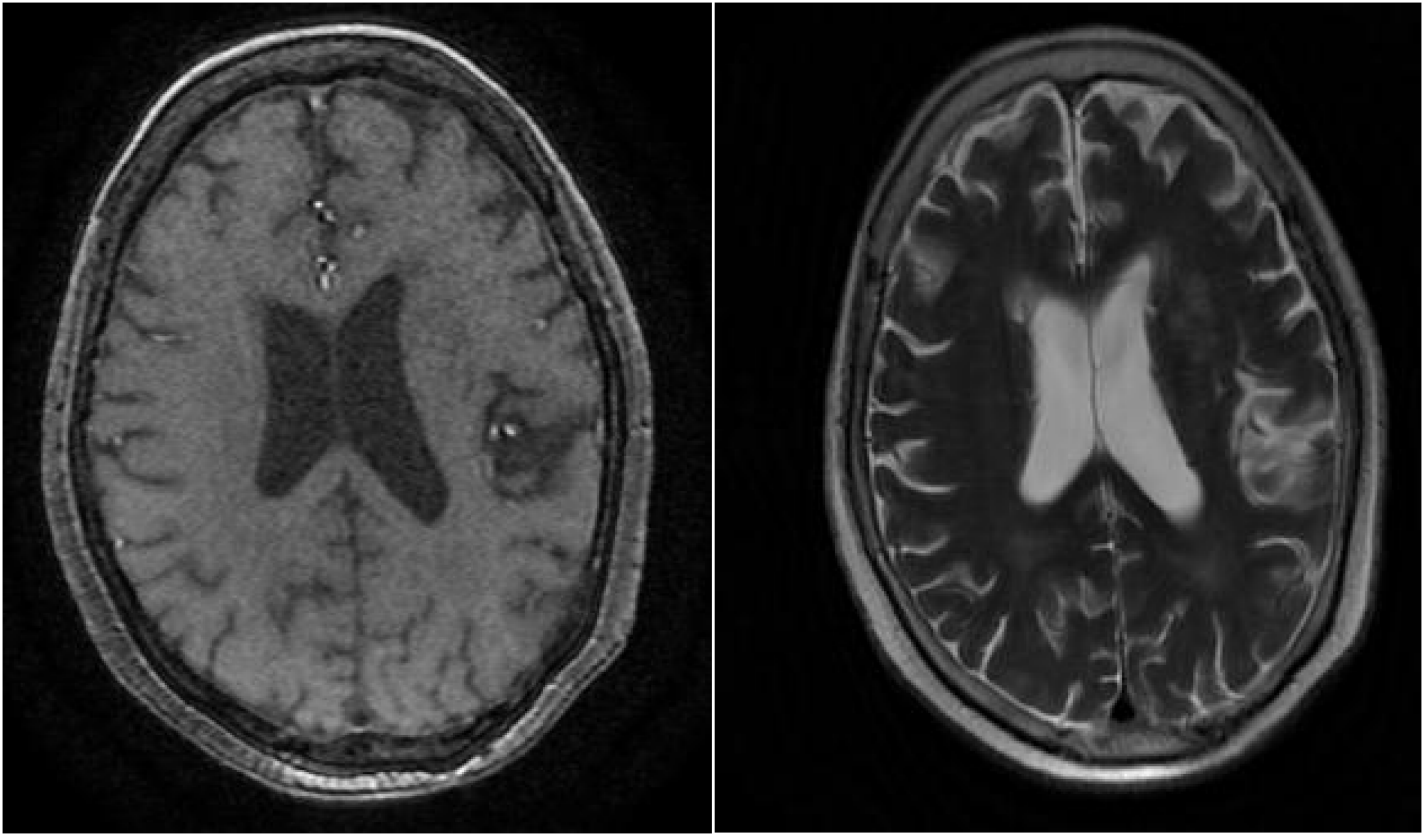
Cranial MRI reveals several punctate low-density lesions in bilateral basal ganglia regions, with slightly deepened and widened cerebral sulci and fissures, centrally located, with no other significant abnormalities observed.
